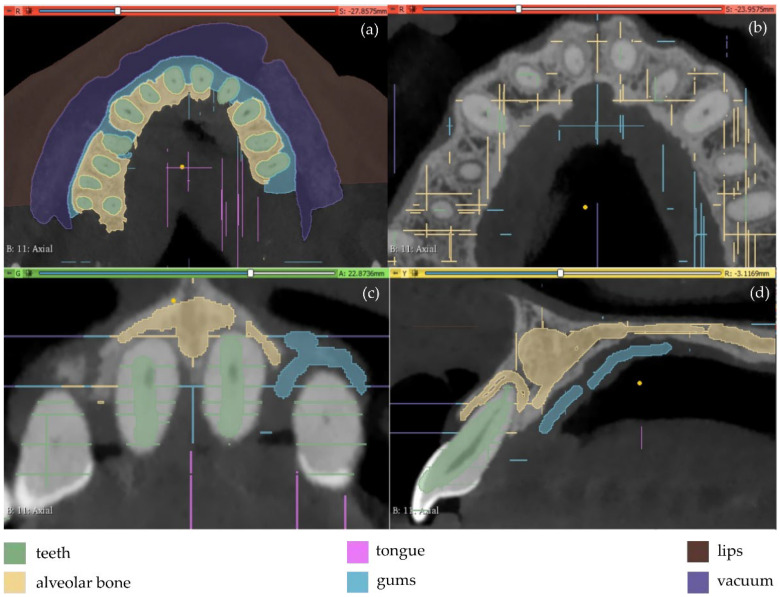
Manual definition of segments in CBCT slices (Experiment 2); (a,b): axial plane; (c): coronal plane; (d) sagittal plane.
